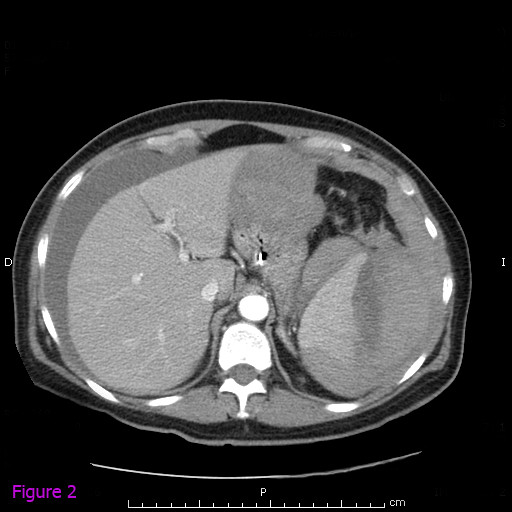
Contrast-enhanced computed tomographic scout view of the abdomen showing splenic subcapsular hematoma with loss of normal structure and normal density of the lower pole, consistent with acute spontaneous splenic rupture.
